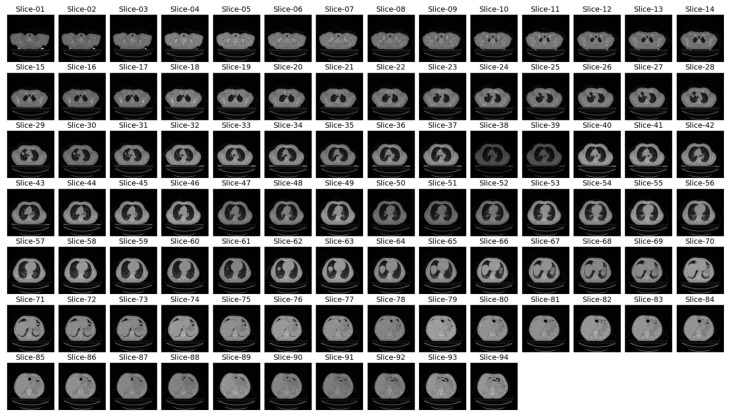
CT slices from patient LUNG1-243 and representation of tumor areas with GTV-1 labeling.
